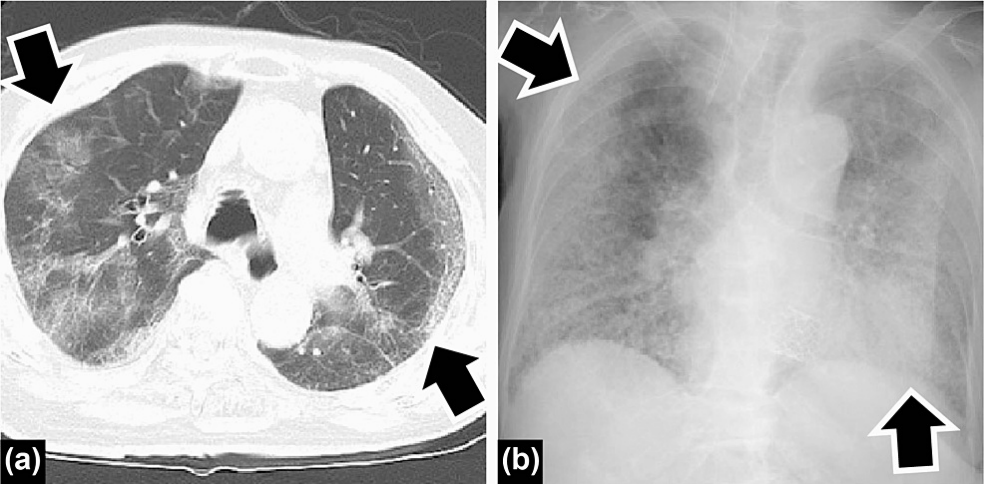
Chest images of the first patient (a) Chest computed tomography on admission. Peripheral predominant ground-glass shadows present in bilateral lung fields; (b) Chest radiography at secondary pneumonia. Diffuse infiltration shadows in bilateral lung fields.
